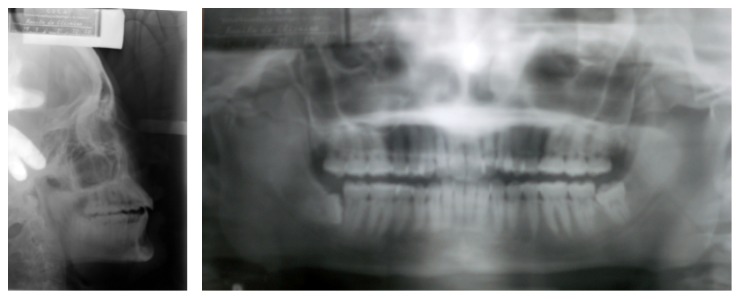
Initial panoramic radiography and teleradiography.
